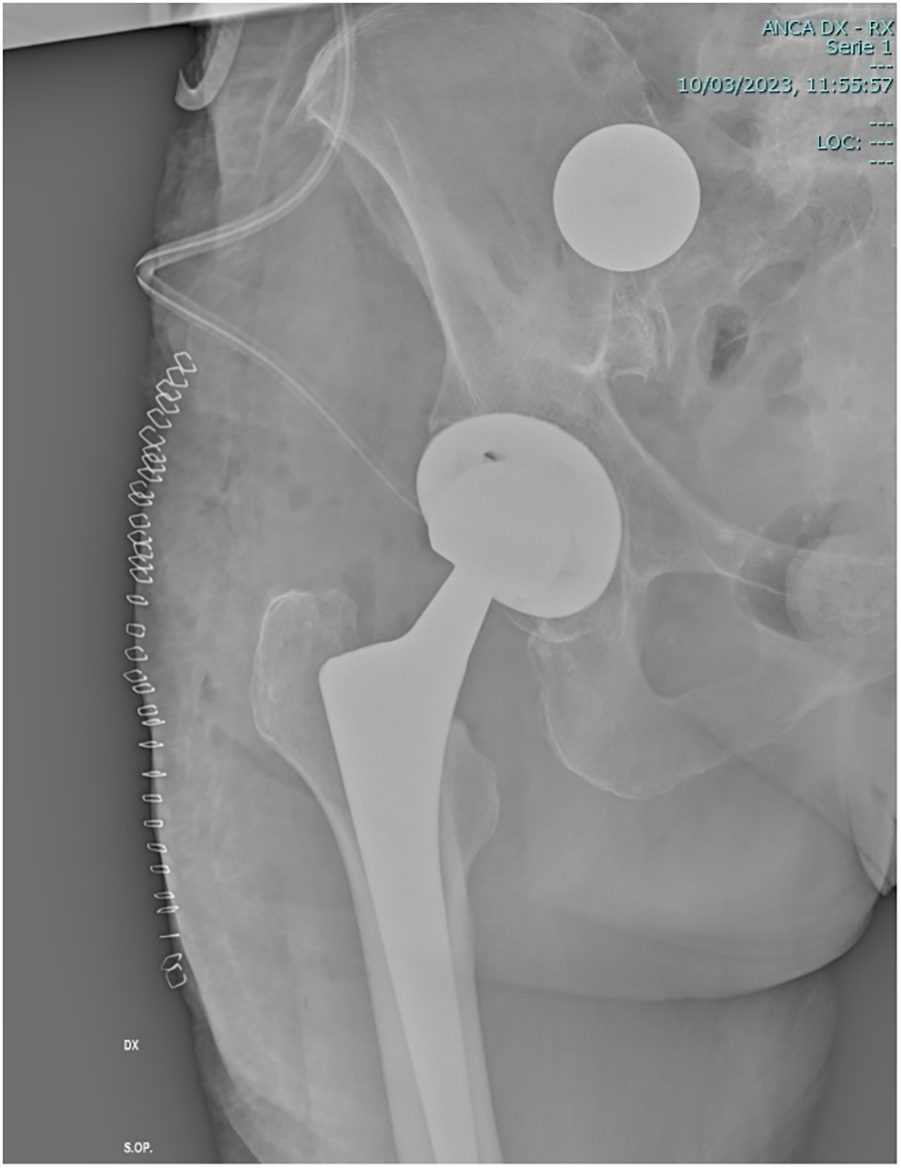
Intraoperative x-ray of the right limb.
